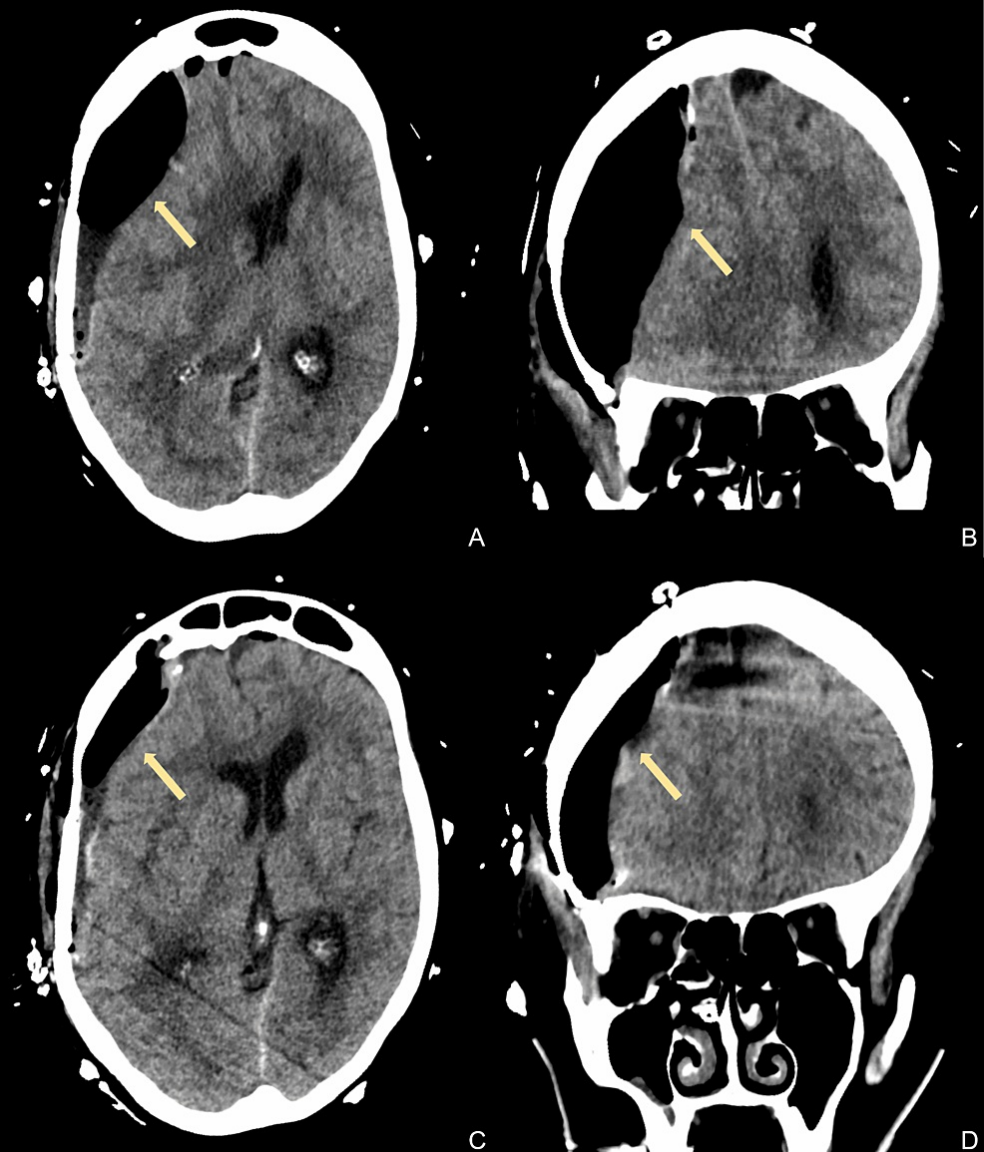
Computed tomography imaging of the head after subdural hematoma evacuation (arrows) The top row shows axial (A) and coronal (B) views on postoperative day 4. The bottom row shows axial (C) and coronal (D) views on postoperative day 4 immediately after subdural evacuating port system placement.
